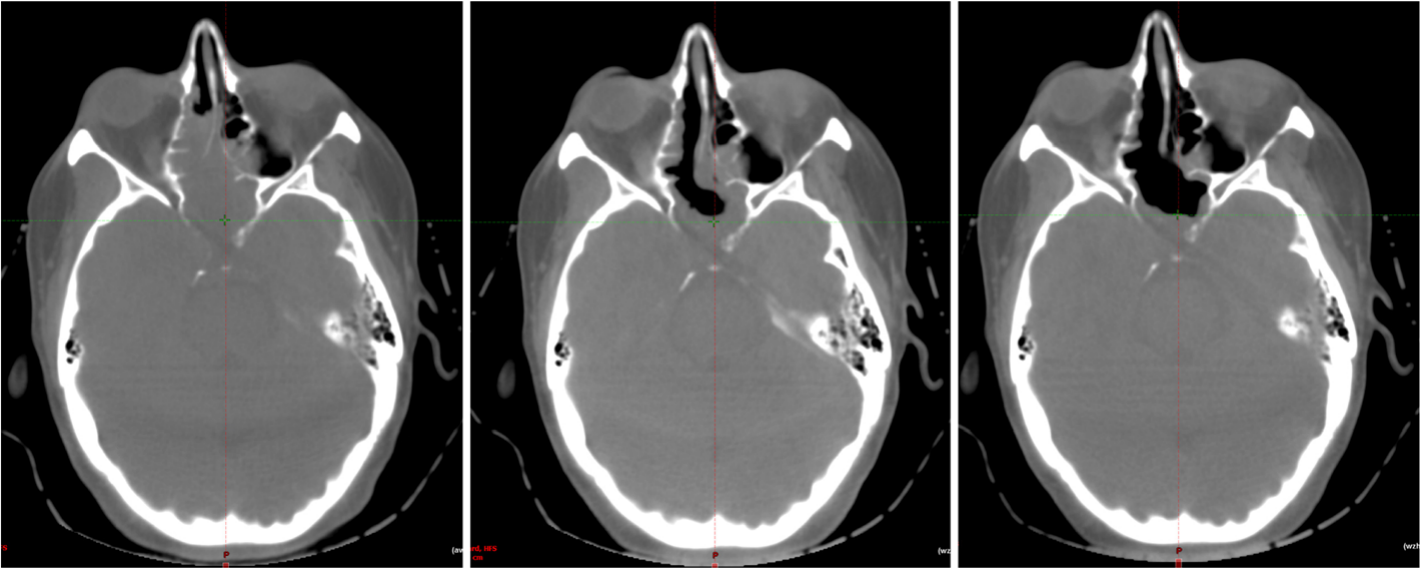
Primary tumor response during radiation therapy as demonstrated by cone-beam computed tomography from first (*left*), tenth (*middle*), and final (*right*) fractions
